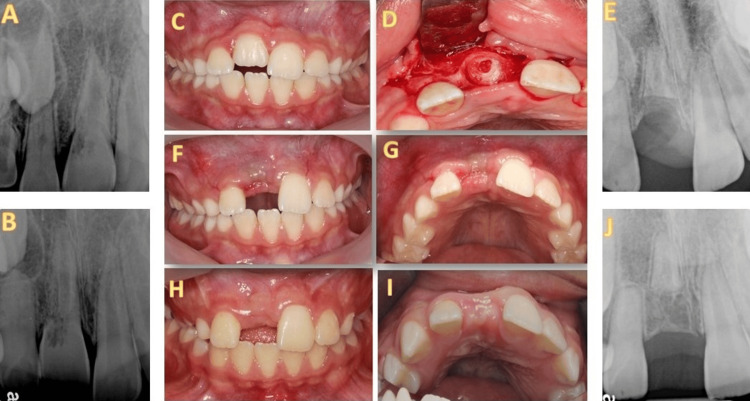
The successful decoronation of an intruded central incisor 30 months years after the treatment (A) Radiograph was taken a year after the intrusion of the upper right central incisor in a 9.5-year-old boy. ECR was observed on the distal aspect of the tooth, Heithersay's class 3. (B) ECR progression after six months. (C) Infraocclusion of the tooth is seen. (D) Photograph was taken during decoronation. The root was reduced to below the bone level, and the canal was allowed to be filled with blood. (E) Radiograph was taken immediately after decoronation. (F) and (G) One week after sutures removal. The flap had been tightly sutured. (H) and (I) Follow up 2.5 years after decoronation. Preservation of the width and height of the ridge can be demonstrated in horizontal (H) and vertical (I) aspects. The horizontal width is partially reduced compared to the adjacent tooth. (J) Radiograph demonstrating the horizontal ridge augmentation 2.5 years after treatment. PDL surrounds the submerged root. Pulp canal obliteration can be observed in the right lateral incisor, which can be related to a previous trauma (not reported).
